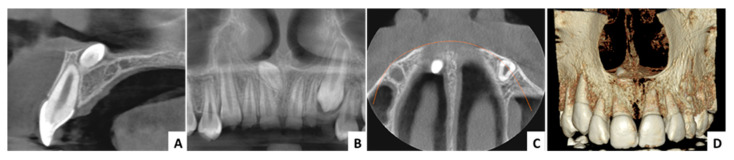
Case 2—radiological aspects: (A) CBCT cross-sectional view, (B)—CBCT panorama view, (C) CBCT axial view, (D) CBCT 3D reconstruction.
